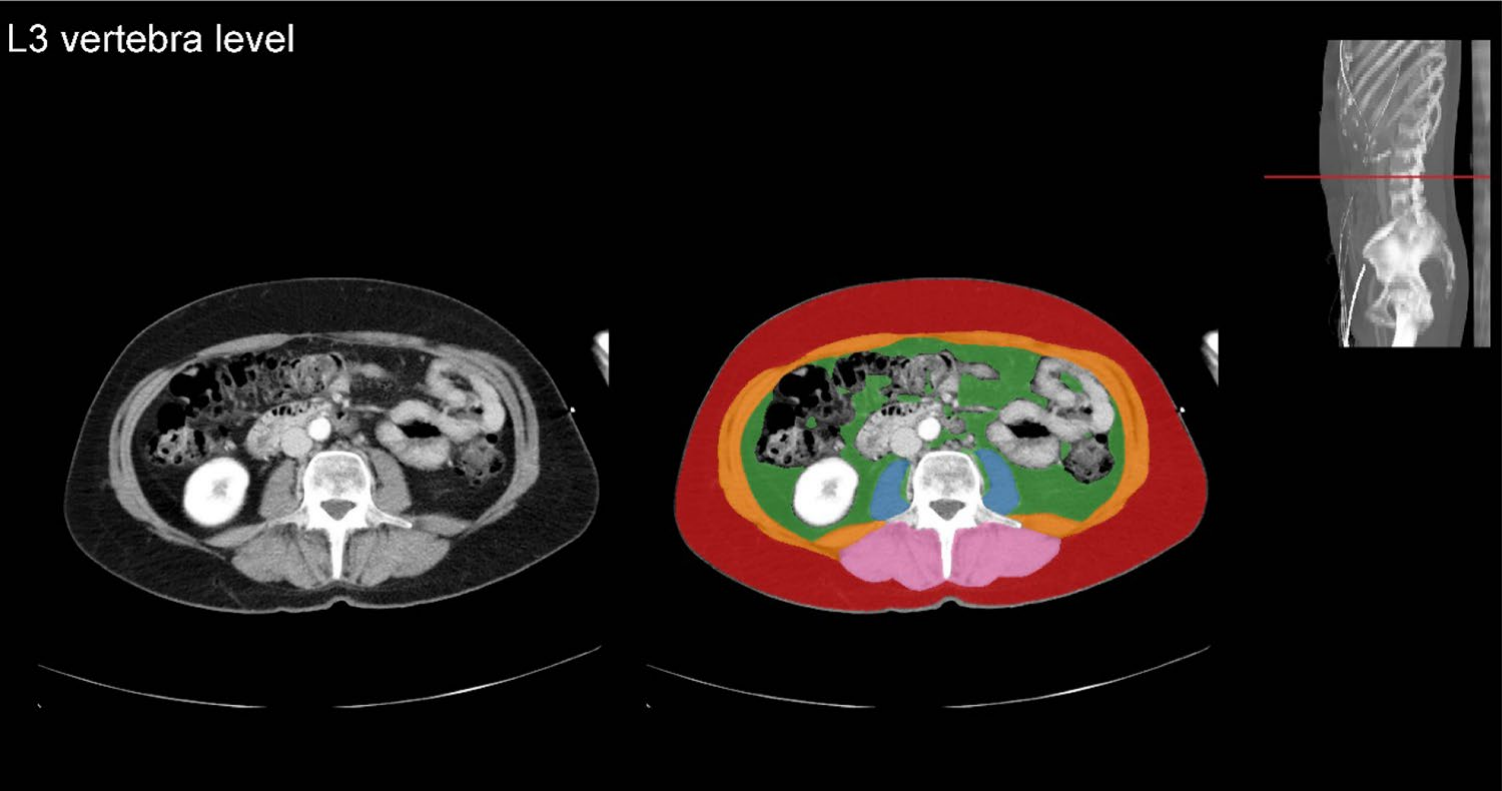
Segmentation of body compositions on an axial computed tomography image
